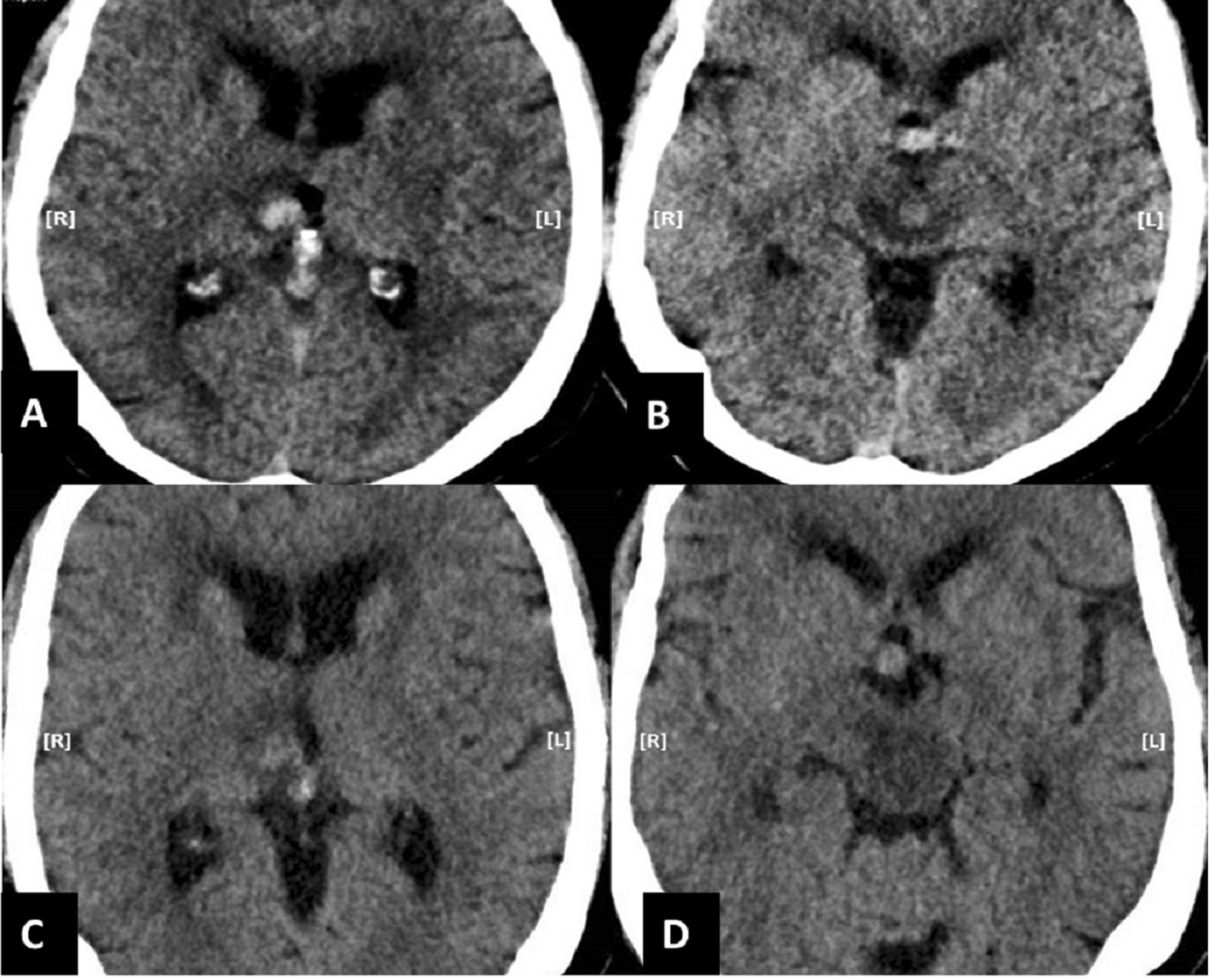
Imaging before conivaptan administration. The immediate post-procedure (intra-arterial stent insertion) CT head (without contrast) demonstrate acute hemorrhage in the right mesial thalamus (A) with surrounding mild vasogenic edema and hypodensity on both sides of mid brain (B). The CT head (without contrast) before conivaptan administration reveals slight decrease in the density of acute hemorrhage in the right mesial thalamus, but with worsening surrounding vasogenic edema (C). The hypodensity in the mid brain has also worsened without any normal density tissue visible, especially adjacent to the quadrigeminal cistern (D).
